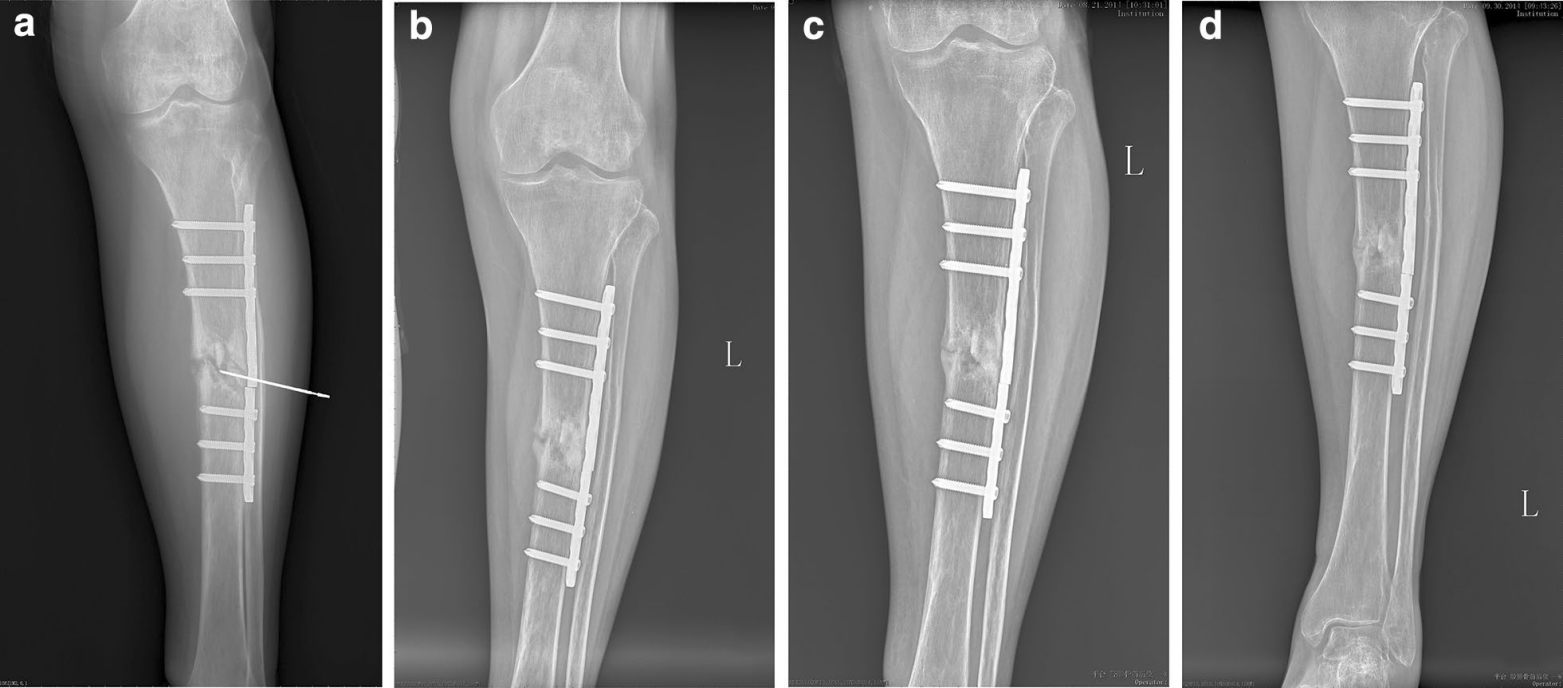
The APL injection and position fixing (a), radiographs of tibia fracture at 2 months (b), 4 months (c) and 6 months (d) after injection
